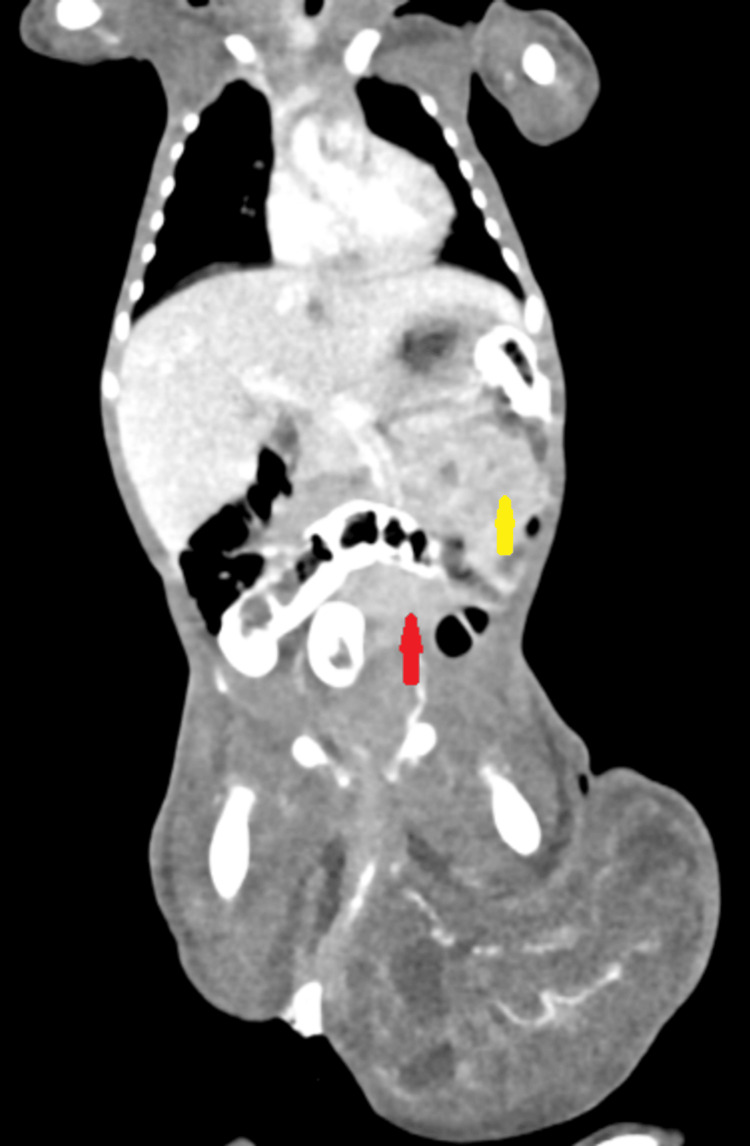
Computed tomography scan, coronal section of the abdomen and pelvis, showing a large solid-cystic mass lesion centered in the coccygeal region with intrapelvic and intra-abdominal extension. Red arrow: Tumor mass with intraabdominal extension. Yellow arrow: Compressed and collapsed small bowel loops.
